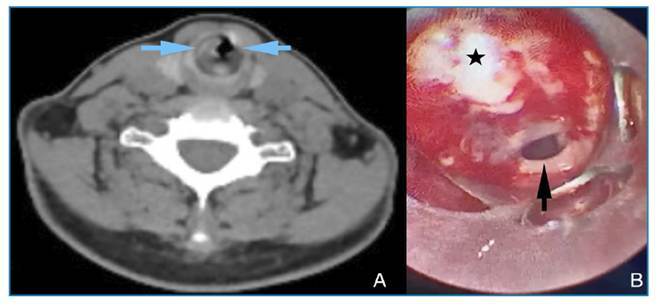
(A) Neck CT image showing heterogeneous increased density circularly surrounding the lumen of the proximal trachea and causing mucosal undulation (blue arrows). (B) Image showing narrowing of the tracheal lumen (black arrow) by fibrinopurulent tissue (black star) circularly surrounding the trachea during rigid bronchoscopy.
